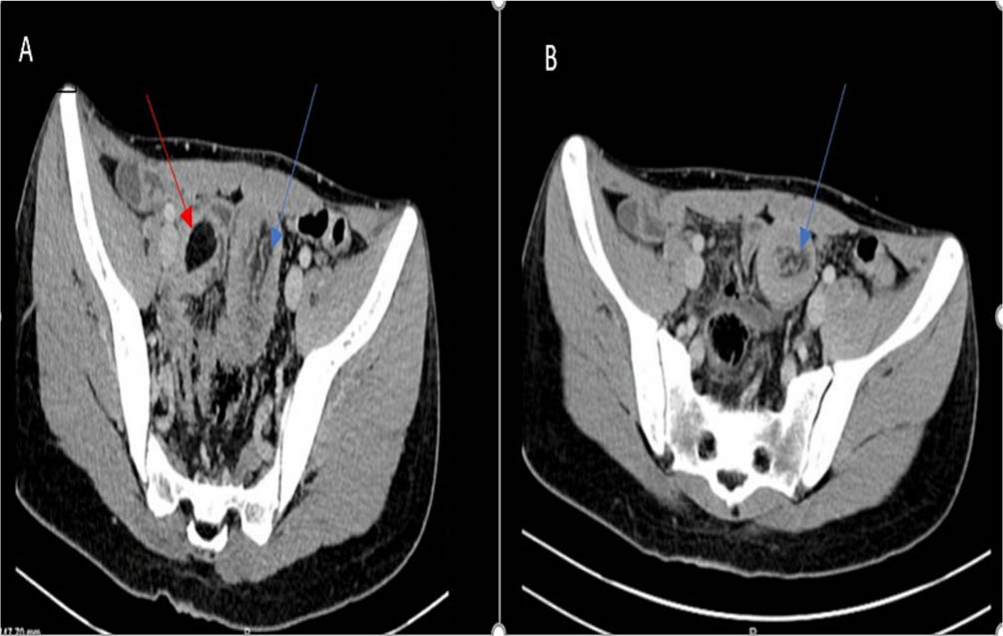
Axial CT scan showing an oval fat density mass representing a jejunal lipoma (A) and a telescoping intestine measuring 20 × 4 cm (B).
